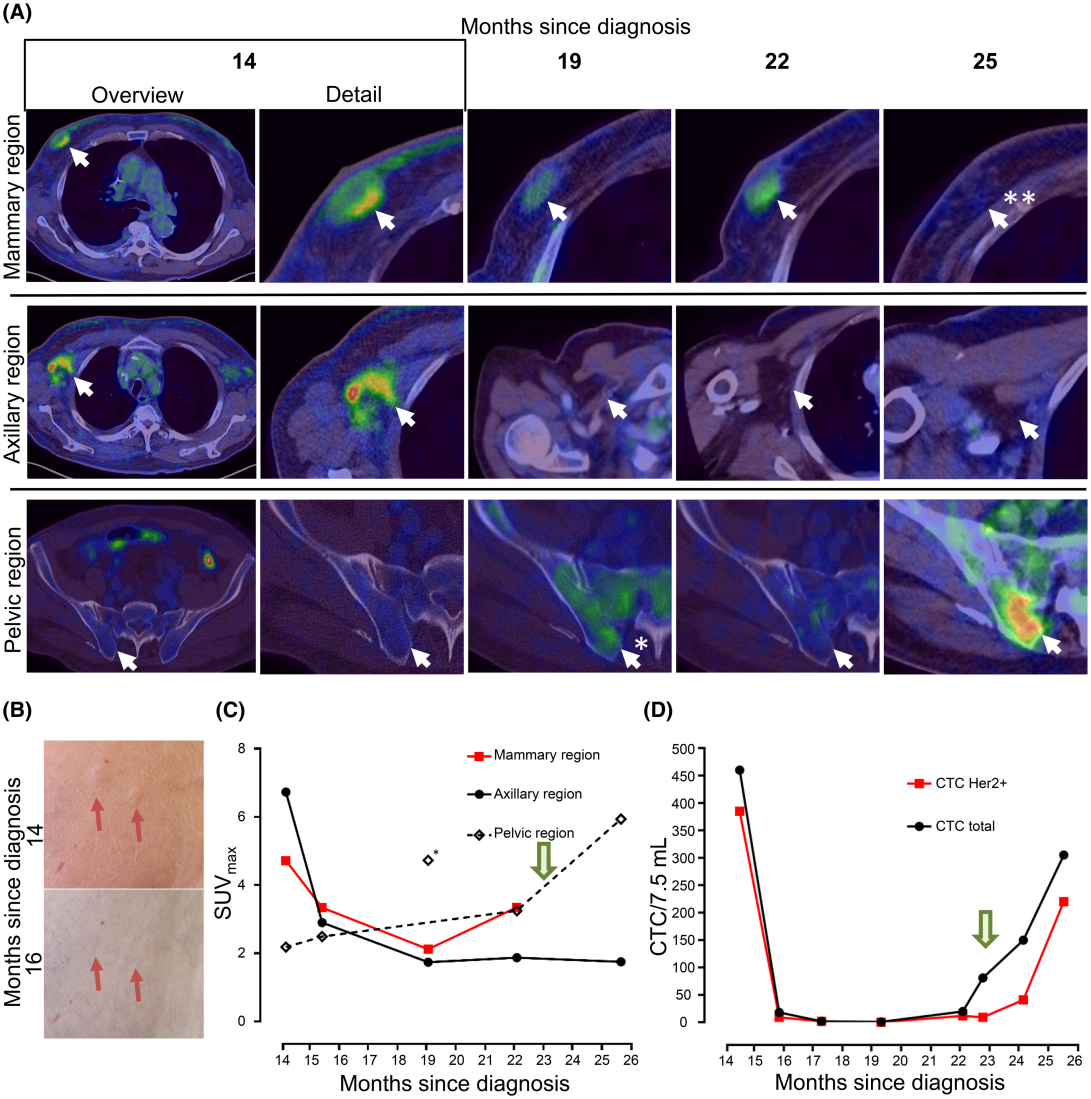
Case presentation, CTC quantification, and PET/CT imaging of patient No.1. (A) Representative PET/CT images from 1st relapse to month 25 at metastatic regions of impact (indicated by an arrow), * indicates high pelvic FDG‐uptake due to unspecific bone marrow activation under therapy,  no standard uptake volume (SUV) measurable after complete resection of mammary metastasis. (B) *Upper panel*: skin metastasis at 1st relapse with intracutaneous nodule accompanied by skin erythema, *lower panel*: regression of skin metastasis 2 months after treatment initiation with Docetaxel, Pertuzumab, Trastuzumab. (C) Course of SUVmax in PET/CT imaging at metastatic regions of impact; * indicates high pelvic SUVmax most likely due to unspecific bone marrow activation under therapy. (D) Course of CTC counts quantified with the CellSearch® system in peripheral blood samples since first relapse; In C and D arrow indicates resection of mammary metastasis. CTC, circulating tumor cell; FDG PET/CT, fluorodeoxyglucose positron emission tomography/computerized tomography; SUV, standardized uptake value.
